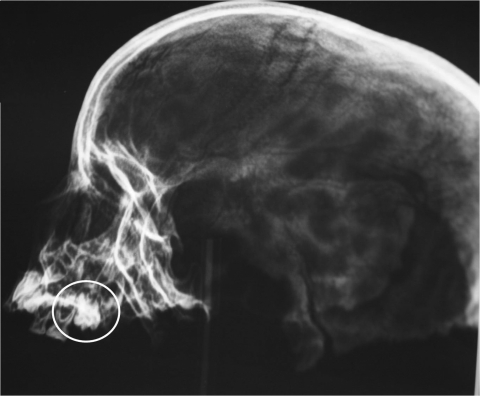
Radiograph of skull found under Sant’ Andrea church in Barletta, Italy, in 2009, showing unerupted teeth (circled) that were later extracted aseptically.
